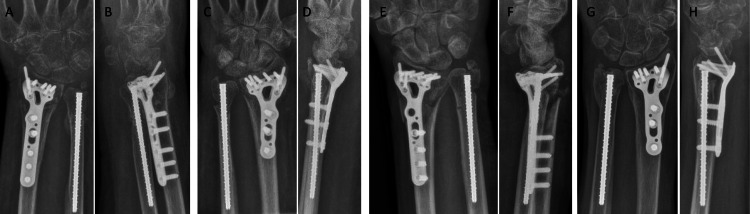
From left to right: 12-week post-operative AP (A,C,E,G) and lateral (B,D,F,H) radiographs of Cases 1 (A,B), 2 (C,D), 3 (E,F), and 4 (G,H), respectively.
